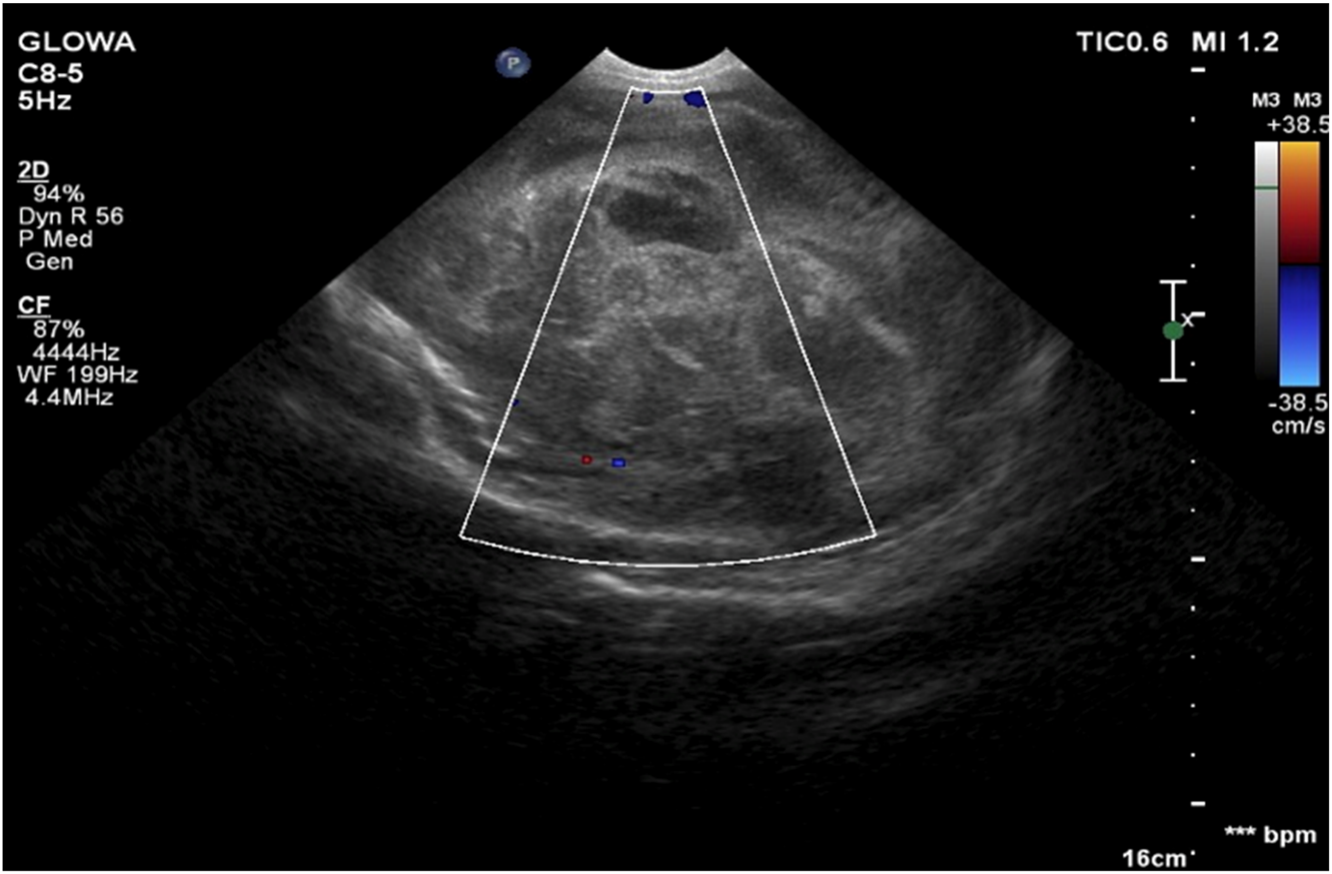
Trans-temporal ultrasound of a newborn with glioblastoma on the lateral plane. Visible lack of flow within the tumor on Doppler examination.
